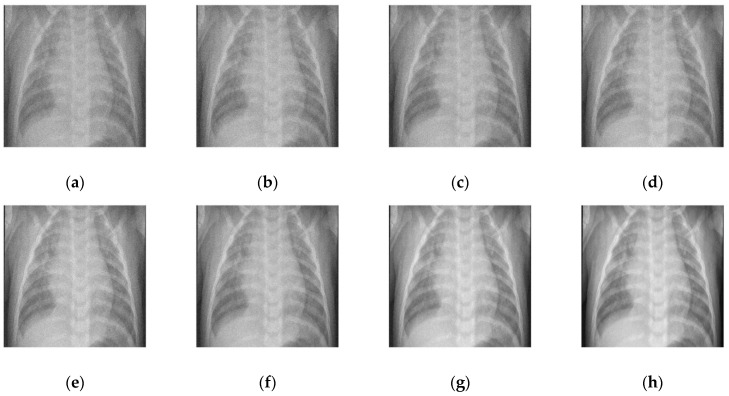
Denoised COVID-19 CT image 4 for the (a) [10], (b) [11], (c) [12], (d) [13], (e) [14], (f) [11], (g) [16], and (h) proposed frameworks, respectively.
